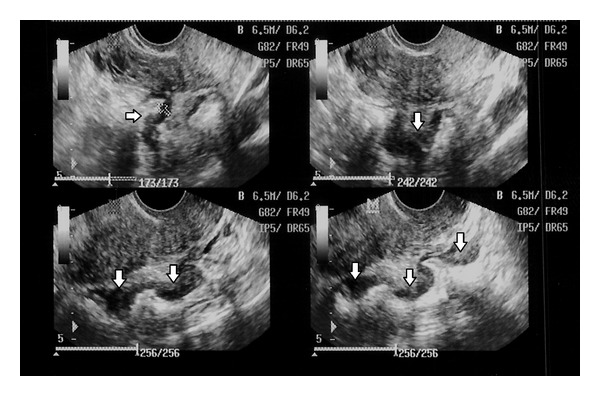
Deeply infiltrative endometriosis lesion of the bowel on ultrasound.
